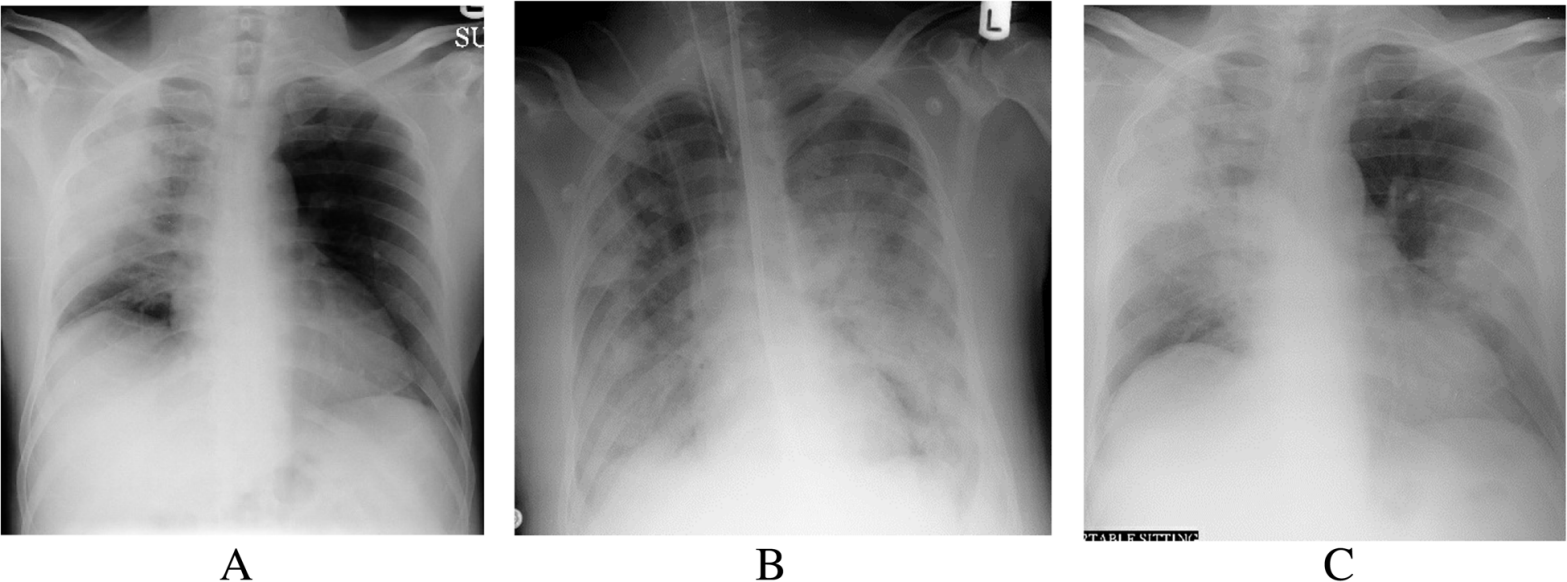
A 65-year-old man with Middle East respiratory syndrome coronavirus. Serial chest radiographs show a type 4 pattern of progression. A A frontal radiograph shows an ill-defined ground-glass opacity occupying the right upper and mid-zone in the peripheral location. B A follow-up radiograph after 3 days shows the bilateral ground-glass type of opacities with a further increase of the right opacity. C A follow-up radiograph after 5 days shows a further extension of the bilateral opacities with the development of consolidation in the left side along with effusion. The patient died on day 8 in the ICU
